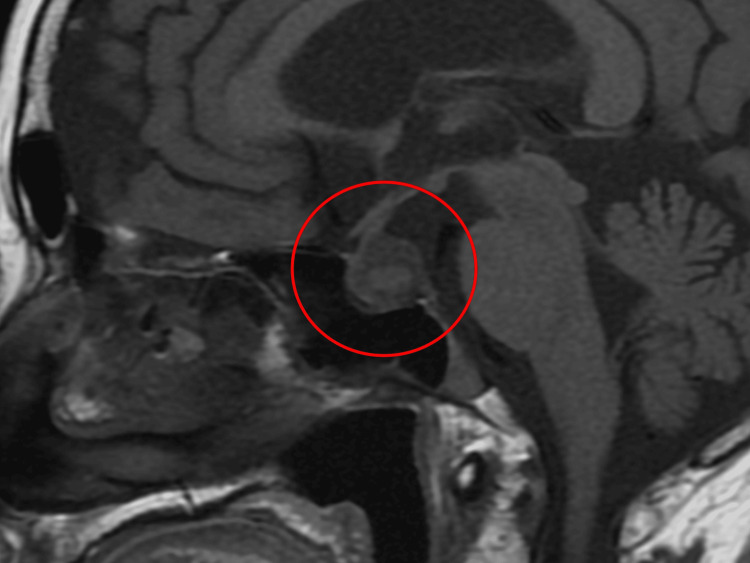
Brain contrast-enhanced magnetic resonance imaging (MRI) revealing a 22-mm cystic lesion in the pituitary gland (red circle)
